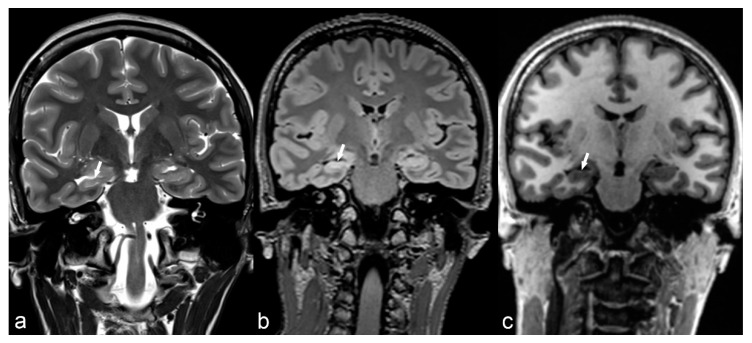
36-year-old woman. The right hippocampus is smaller than the left (arrows) as seen on coronal T2WI (a), FLAIR (b) and MP2RAGE (c) and shows hyperintensity on T2WI and FLAIR. There is also flattening and loss of the normal undulations of the right hippocampus, suggestive of hippocampal sclerosis.
